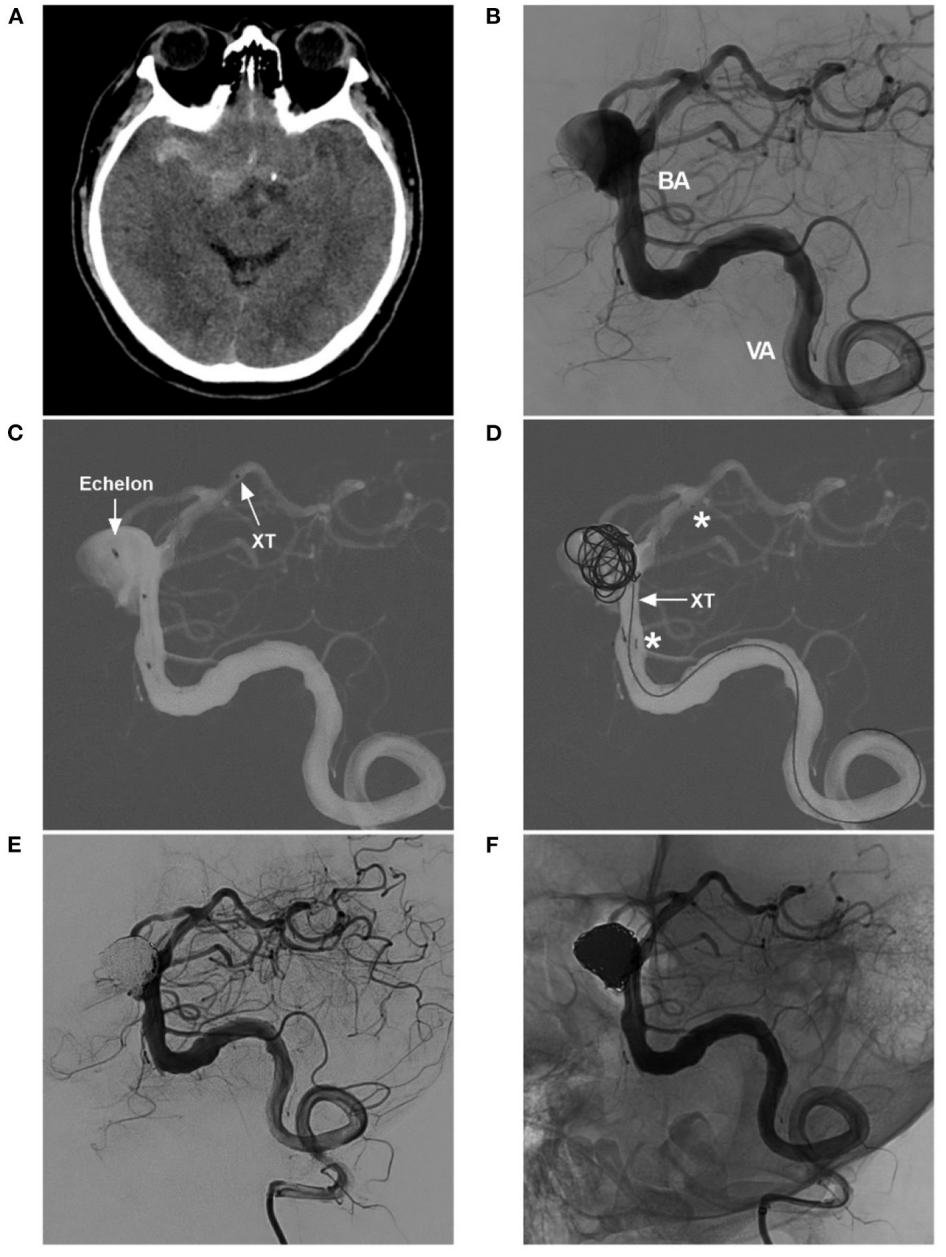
Atlas stent-assisted coiling of an aneurysm in VBD. (A) CT showing subarachnoid hemorrhage. (B) Two-dimensional DSA showing the VBD and an aneurysm of the BA. (C) Road map showing the Echelon-10 microcatheter (Medtronic, Irvine, California, USA) in the aneurysm (arrow with Echelon). The XT-17 microcatheter tip (arrow with XT) (Stryker Neurovascular, Fremont, California, USA) crossed the aneurysm neck and entered the posterior cerebral artery. (D) Road map showing an Echelon-10 microcatheter jailed in the aneurysm, which delivered the coils. Then, Atlas stent was deployed; the asterisks indicate the proximal and distal markers. Then, the XT-17 microcatheter (arrow with XT) was guided into the aneurysm by the transcell technique. (E,F) Two-dimensional unsubtracted (C) and subtracted (D) DSA showing that the aneurysm was coiled completely. BA, basilar artery; CT, computed tomography; DSA, digital subtracted angiography; VA, vertebral artery; VBD, vertebrobasilar dolichoectasia.
